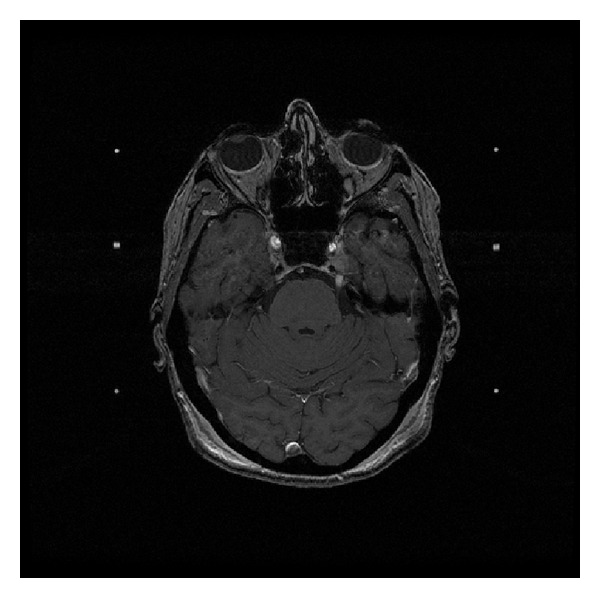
Axial T1 postgadolinium enhanced MRI showing the lesion of the left trigeminal nerve at the time of its original diagnosis as trigeminal schwannoma.
